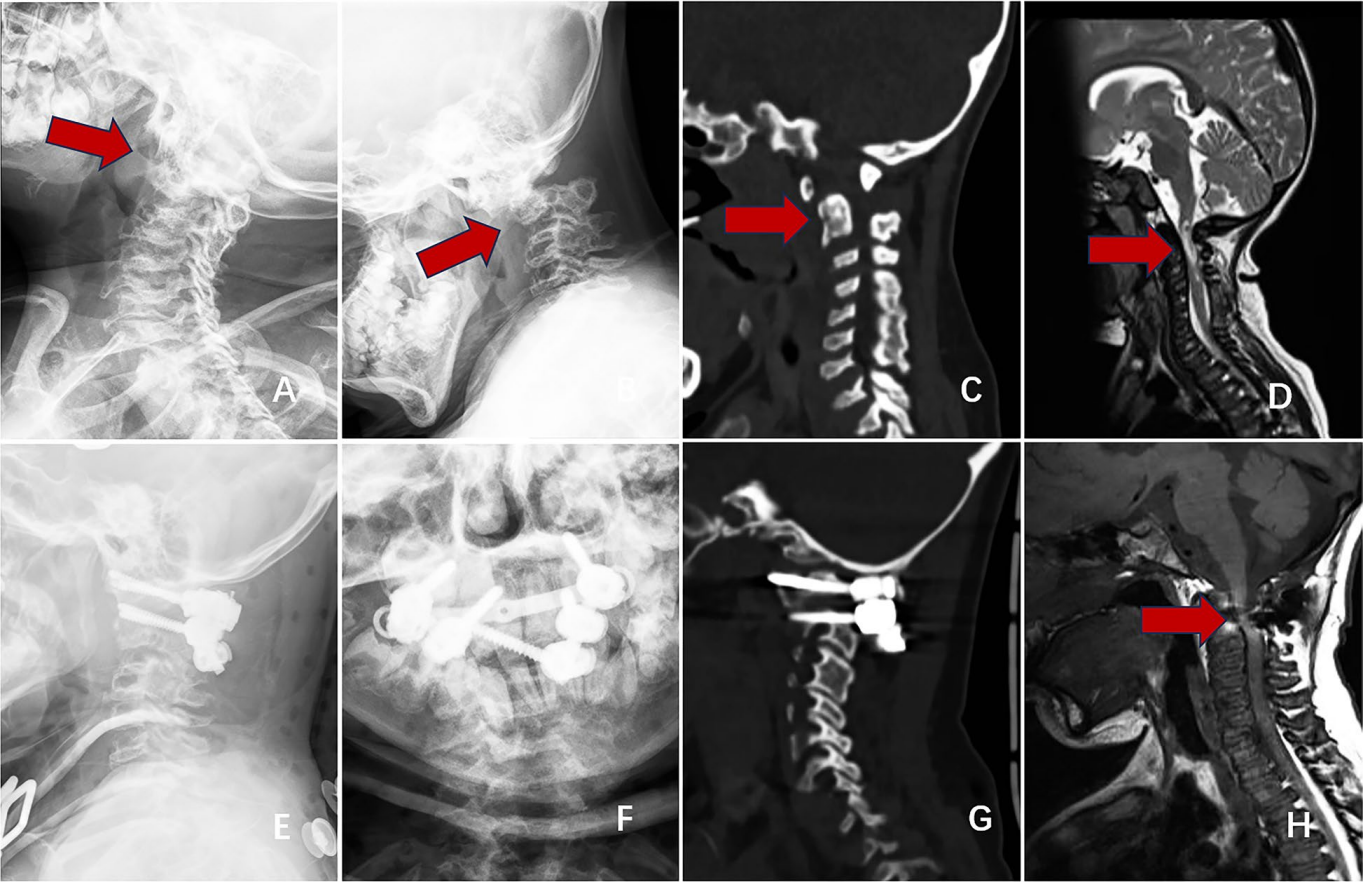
Imaging findings of an 8-year-old patient who was admitted for atlantoaxial dislocation, spinal stenosis, and spinal cord compression and subsequently underwent surgery. Preoperative dynamic radiographs indicating atlantoaxial instability and dislocation (A, B). Preoperative CT image showing the absence of the odontoid process and atlantoaxial dislocation (C). Preoperative MRI indicating cervical spinal stenosis and spinal cord compression (D). Postoperative radiography and CT showing successful atlantoaxial reduction and accurate screw insertion (E, F, G). Postoperative MRI showing relief of the cervical spinal cord compression (H)
